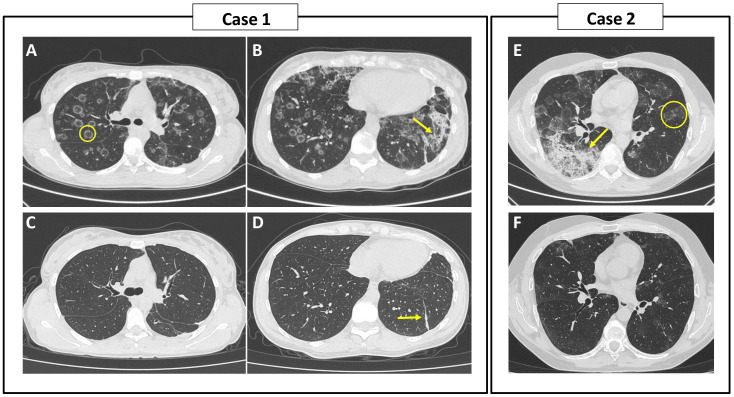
Case 1: multiple bilateral rounded ground-glass opacities with reversed halo sign [(A)-circle], associated with a bandlike subpleural consolidation at the left lower lobe [(B)-arrow]. Ground glass opacities showed random distribution (both central and peripheral), without cranio-caudal predominance (upper or lower lobes). No other extra-parenchymal ancillary findings (such as pleural effusion or lymphadenopathies) were detected. After corticosteroid therapy, complete regression of atoll sign (C), with residual fibrotic bundle in the left lung base (D). Case 2: wide consolidation at the apical segment of the right lower lobe [(E)-arrow], associated with multiple bilateral ground glass opacities with atoll sign [(E)-circle]. After therapy, complete regression, with residual mild ground-glass (F). All CT scans were performed using a 128-slice CT scanner (Ingenuity; Philips) before and after contrast media injection, with the following technical parameters: tube voltage, 120 kV; tube current modulation, 100–250 mAs; spiral pitch factor, 1.224; collimation width, 64 × 0.625. For lung parenchyma evaluation, high-resolution reconstructions were obtained using a convolution kernel (Y-SHARP) at a slice thickness of 1 mm.
